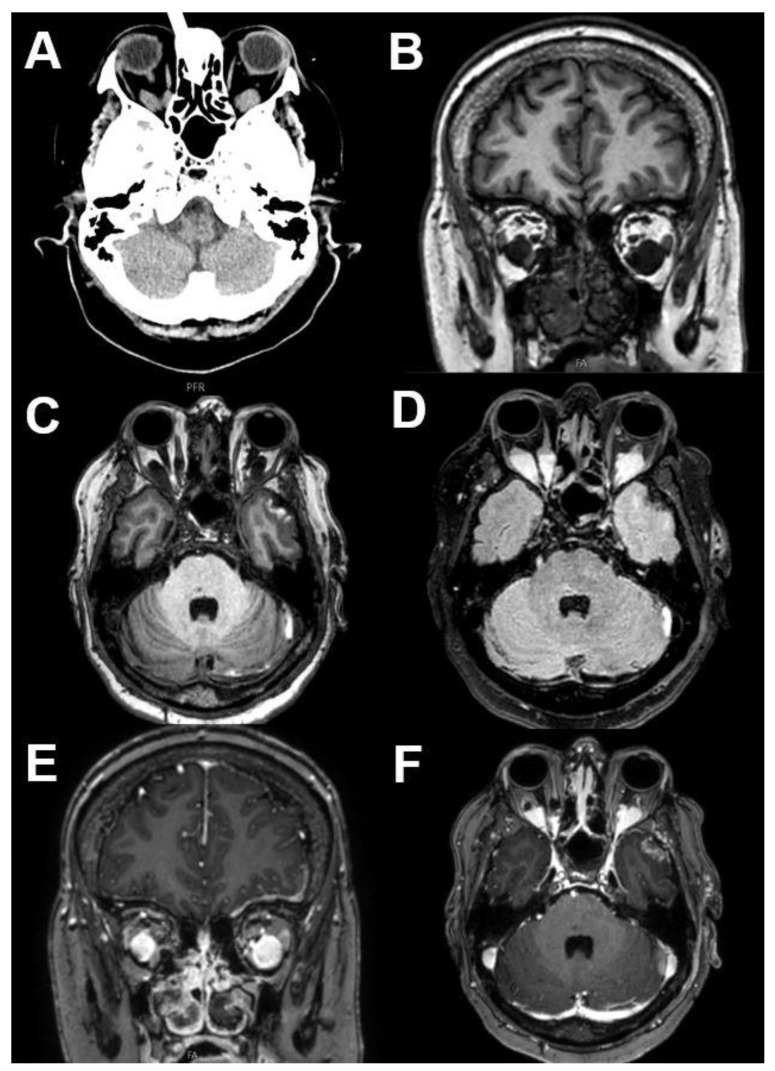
Venous varices.* CT and MRI of a patient with bilateral orbital venous varices. CT shows bilateral dorsal orbital mass isodense to intraorbital muscles (A). Varices show T1-hypointense (B,C) and FLAIR-hyperintense signal (D). After contrast media application a marked enhancement can be observed (E,F).
